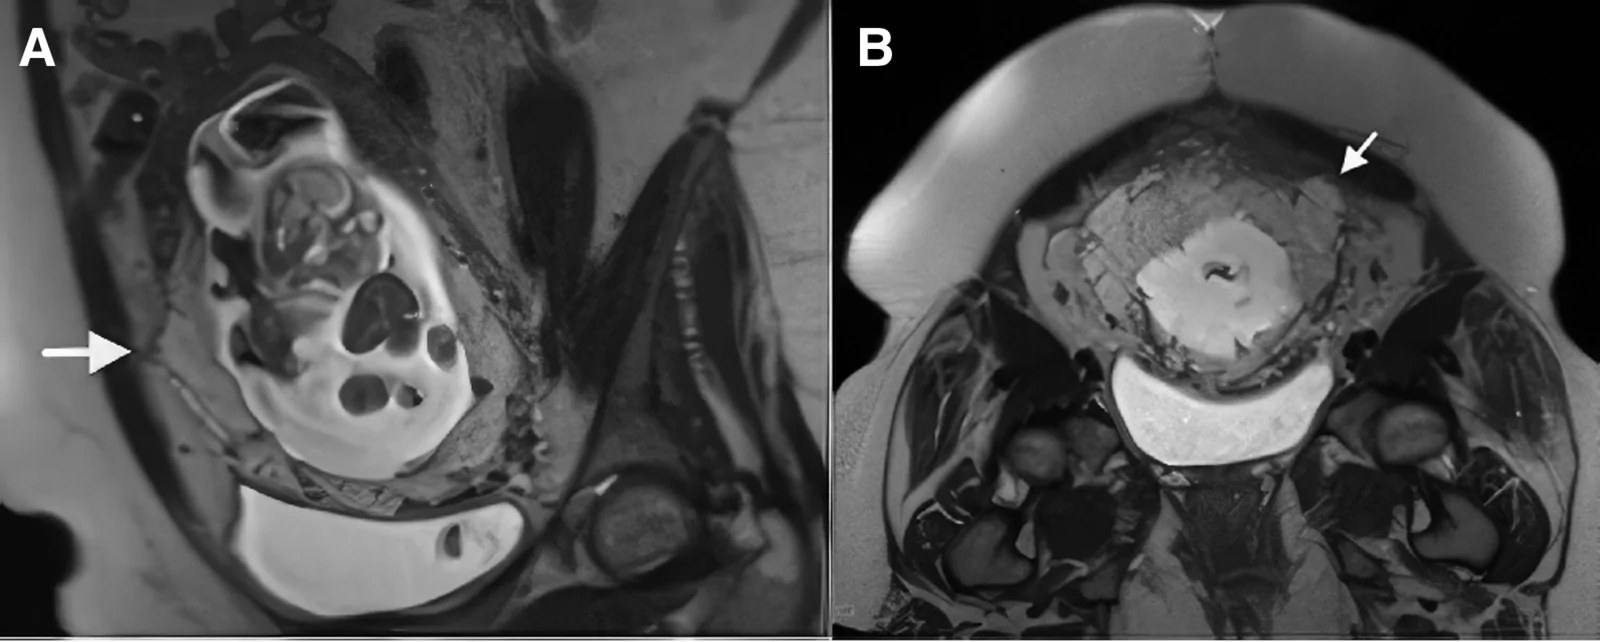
MRI demonstrates placental invasion into the myometrium with external protrusion. (A) Sagittal view of the uterus showing placental implantation. (B) Transverse view of the lower uterine segment. MRI = magnetic resonance imaging.
